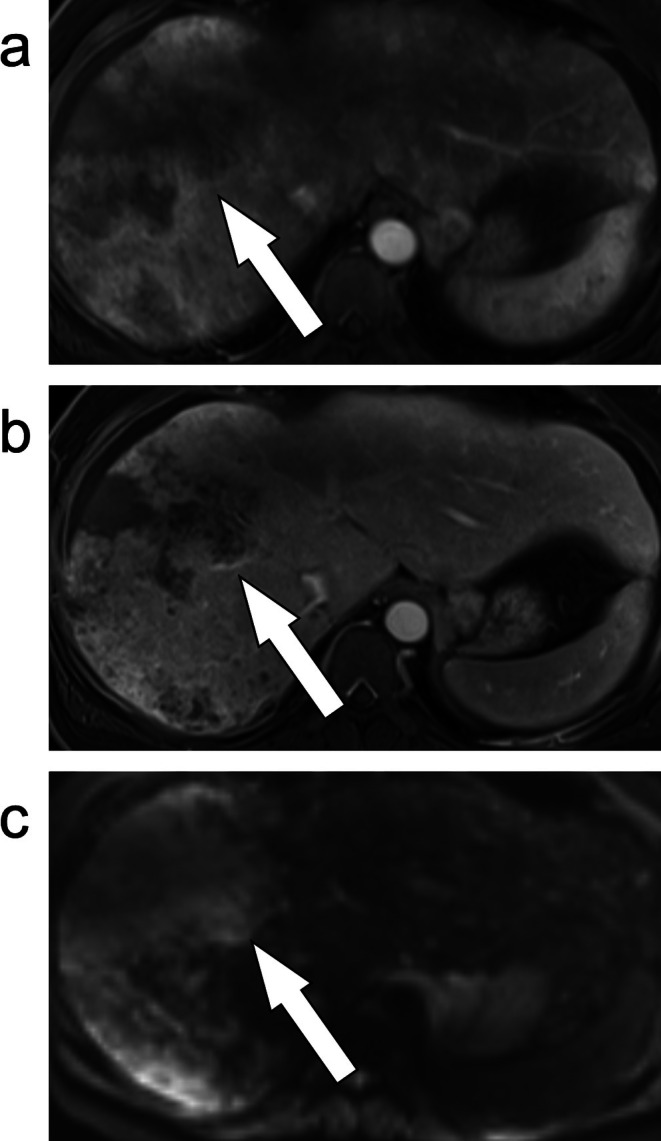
Axial arterial phase (a) and portal venous (b) phase post‐contrast T_1_‐weighted fat‐suppressed MR images, as well as diffusion weighted imaging (c) demonstrate an infiltrative appearance of an intrahepatic cholangiocarcinoma categorized as LR‐M (arrows). Infiltrative HCC can appear similarly.
